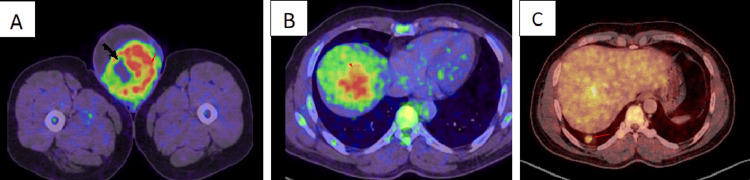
Axial post-processed fused PET-CT shows avid FDG uptake (A) Axial post-processed fused PET-CT shows avid FDG uptake within the solid cystic lesion of the left hemiscrotum (short arrow), (B) FDG-avid delayed-enhancing lesion in segment 7 of the liver (arrowheads), and (C) the largest nodule with FDG uptake in the right posterobasal segment (long arrow). PET/CT: positron emission tomography/computed tomography, FDG: fluorodeoxyglucose.
